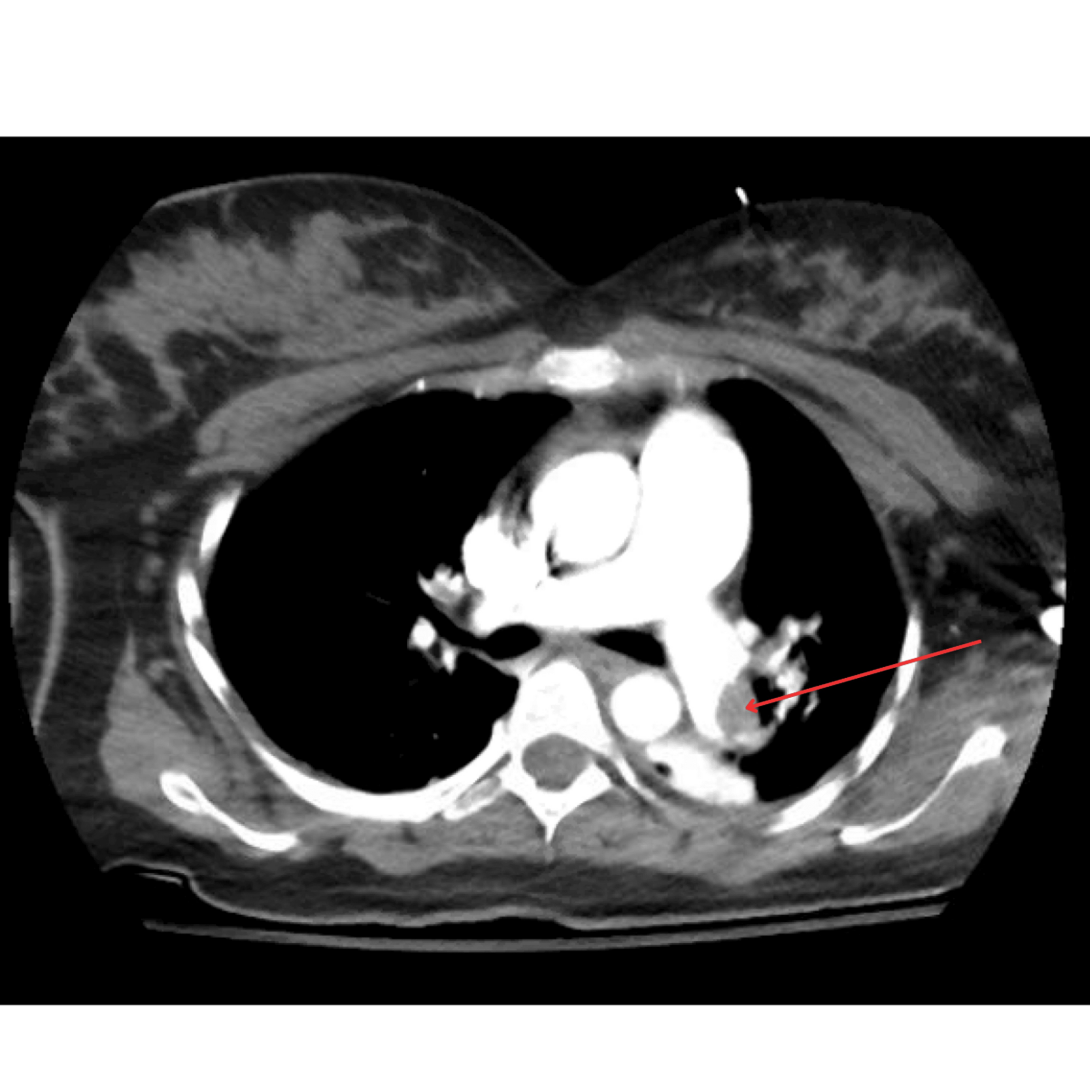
CTA chest pulmonary embolism CTA: computed tomography angiography Demonstrating embolus in the left distal main pulmonary artery
